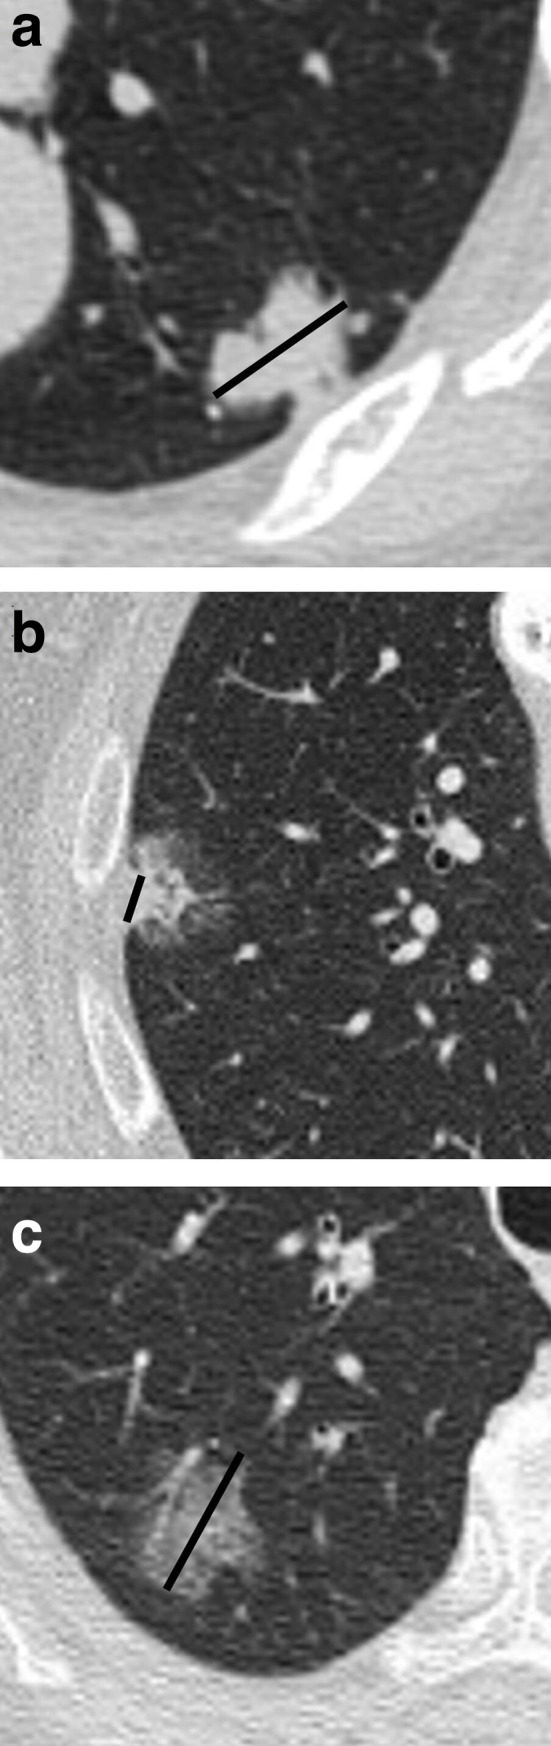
Axial non-enhanced CT showing (a) a solid nodule in the left lower lobe compatible with a biopsy-proven invasive adenocarcinoma. It is recommended that the solid part be measured (black line). The solid part represents the invasive component of the tumour and should be used to define the T classification for staging. In (b), a part-solid tumour in the right upper lobe is shown. It is recommended that the solid dimension (black line) of the tumour for the T-descriptor be used. In (c), a pure ground-glass nodule is shown. The largest dimension of the ground-glass tumour should be measured and should be classified as an adenocarcinoma *in situ* (cTis).
